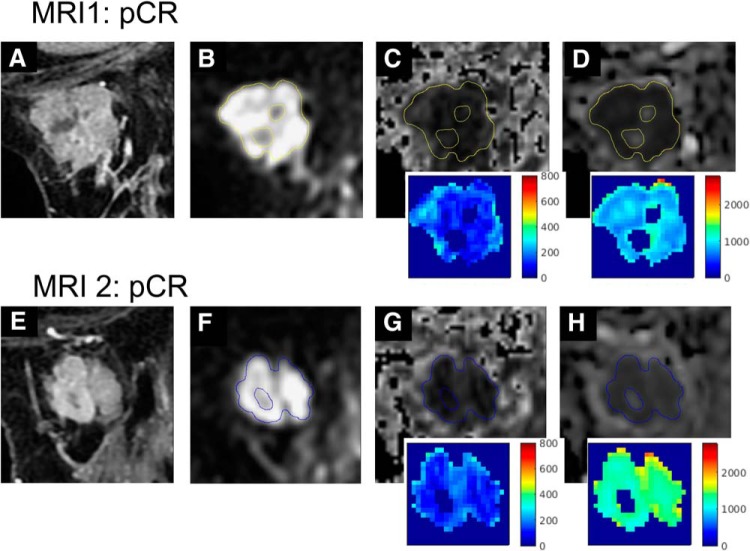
Example images from a patient with locally advanced breast cancer (LABC) who was categorized as pCR based on postsurgical pathology. Dynamic contrast-enhanced magnetic resonance imaging (DCE-MRI) subtraction images (precontrast subtracted from early postcontrast) and diffusion tensor imaging (DTI) images (b = 600, FA map, and ADC map) are shown for the pretreatment time points (A, B, C, and D) and early treatment time points (E, F, G, and H). The tumor region of interest (ROI) is indicated by the contour line on the DTI b = 600 images and FA and ADC maps, and colorized maps of the FA and ADC values within the tumor ROI are also shown.
